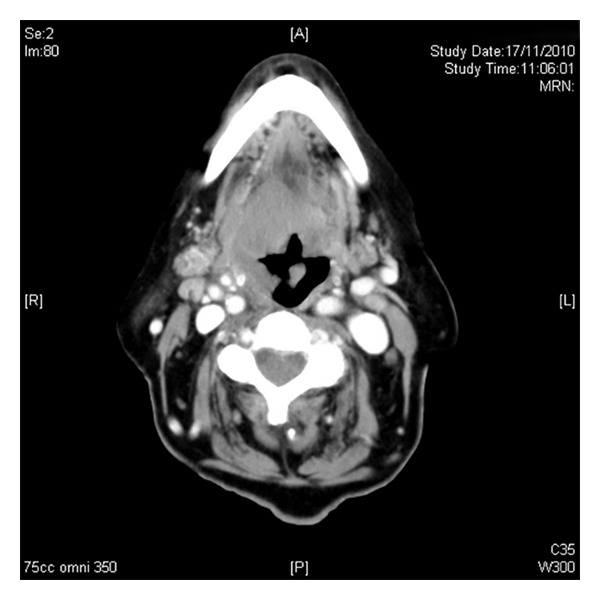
Computed tomography at presentation.
